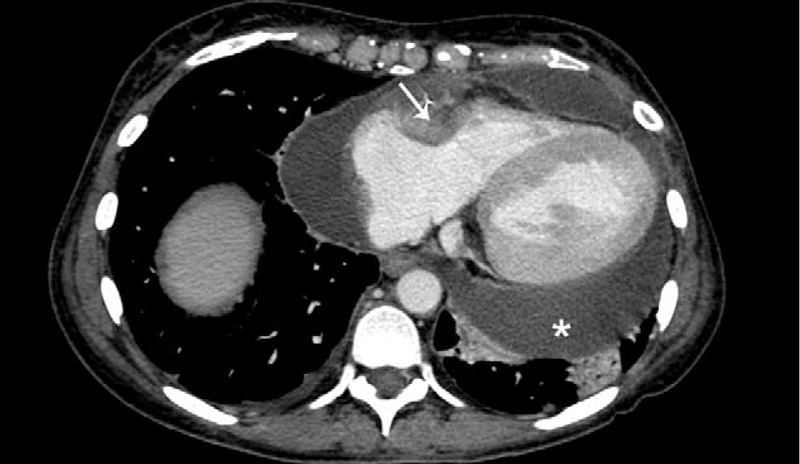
Axial contrast-enhanced computed tomography scan image of the chest showing pericardial effusion (^∗^) and soft tissue involving right atrioventricular groove, extending to right ventricular free wall and right atria (arrow).
